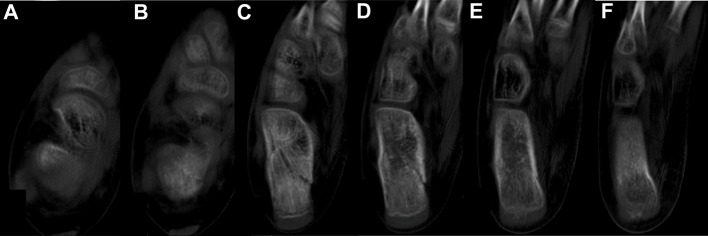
Computed tomography images demonstrate: (A, B) axial slices showing the end of the subtalar joint; (C-F) sequential axial slices from proximal to distal showing the fracture of the calcaneal body and not showing articular fracture.
